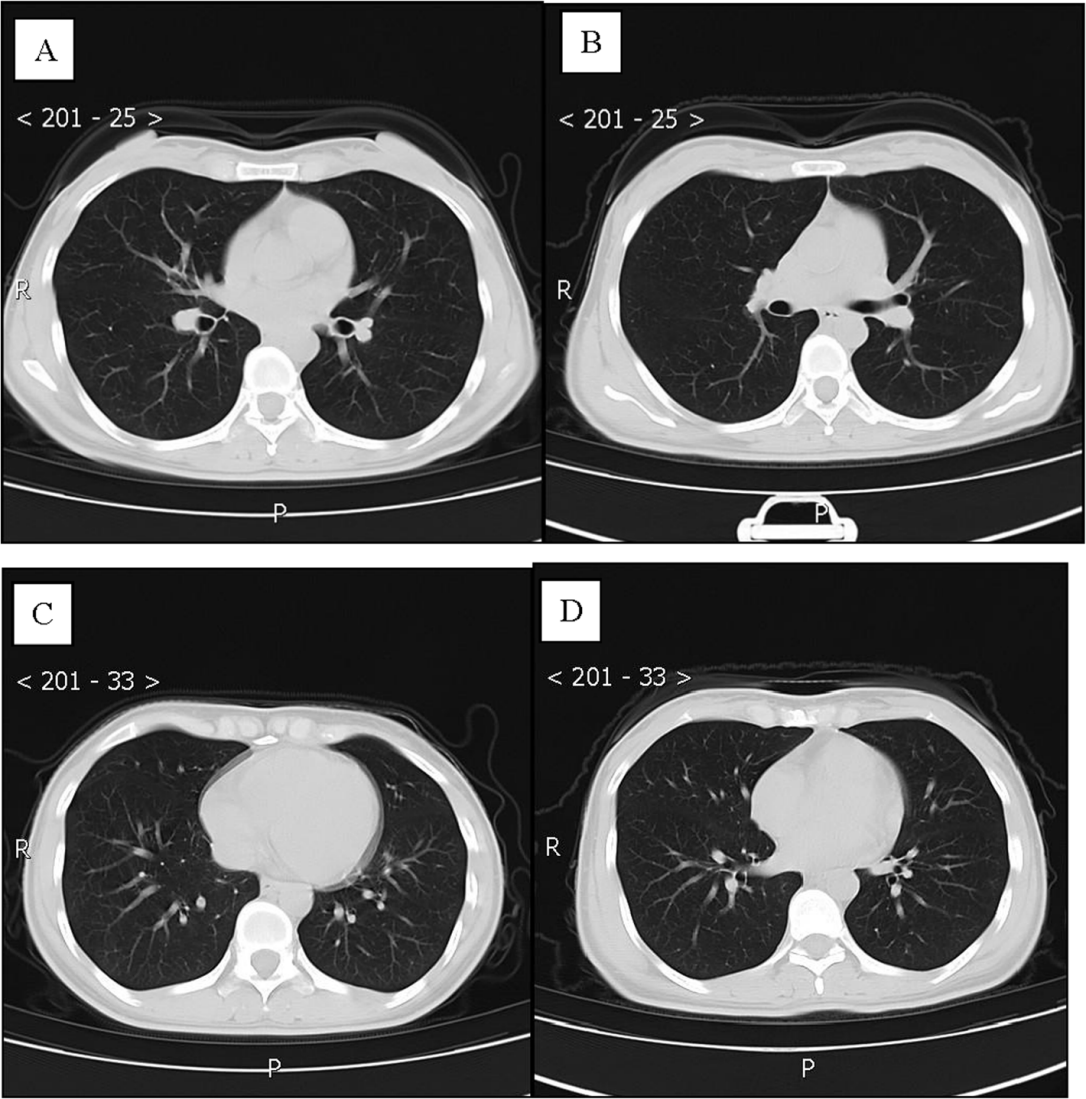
Chest CT images of A 38-year-old woman with COVID-19 infection. a, c Transverse chest CT images showed no abnormalities in both lungs on admission. b, d Transverse chest CT images showed no abnormalities in both lungs on discharge
